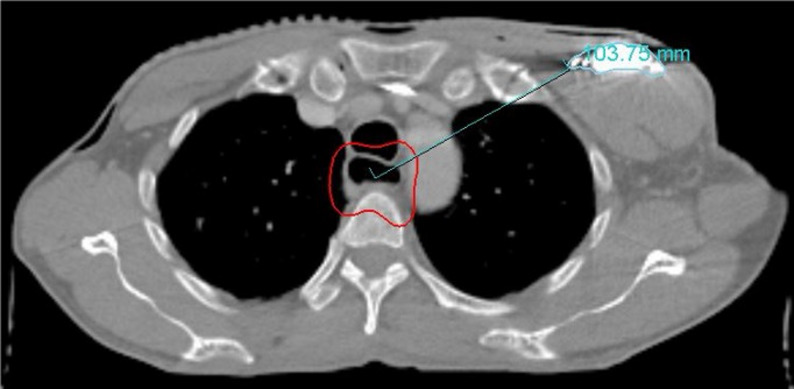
Shows an axial CT image depicting the measurement of distance from the geometric centre of the PTV (outlined in red) to the surface of the implanted pacemaker device. Primary endpoints for the CIED were Dmax (point), D0.03 cc, and Dmean. Secondary endpoints included organ-specific dose metrics, standard plan quality indices (PTV D95, conformity index, HI), and efficiency (monitor units and beam-on time). All DVHs were exported at a resolution of 0.01 Gy. Plans were considered acceptable only if PTV coverage and significant OAR limits were met
